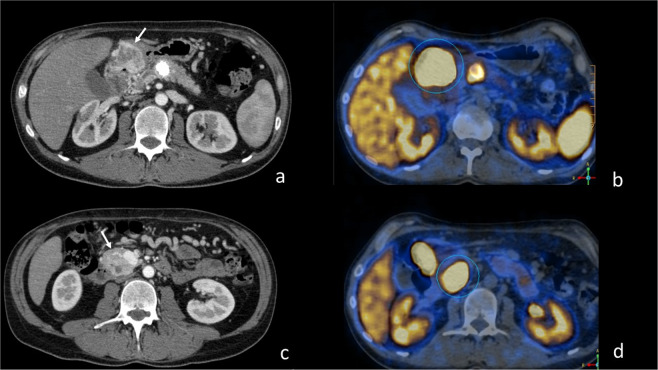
CT scans of multifocal PanNET inhomogeneous hyperdense in arterial phase (a, c) due to hypervascularization and increased uptake of ^68^Ga-DOTATOC PET/TC (circle in b and d) involving the stomach (arrow in a), the uncinate process of the pancreas (arrow in c) and the pancreatic body with an extensively calcified mass
